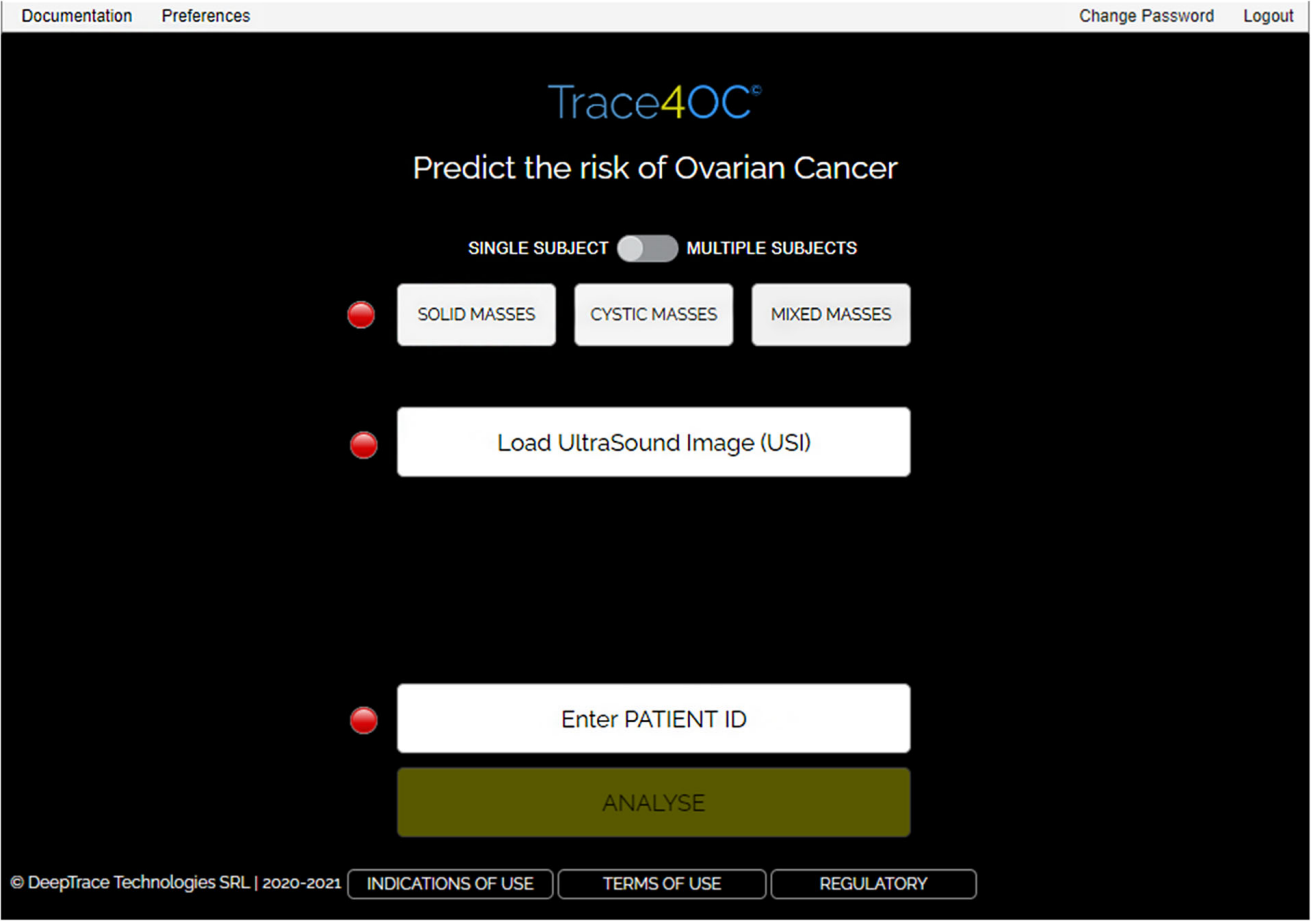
Software interface for the mass types and transvaginal ultrasound image selection
